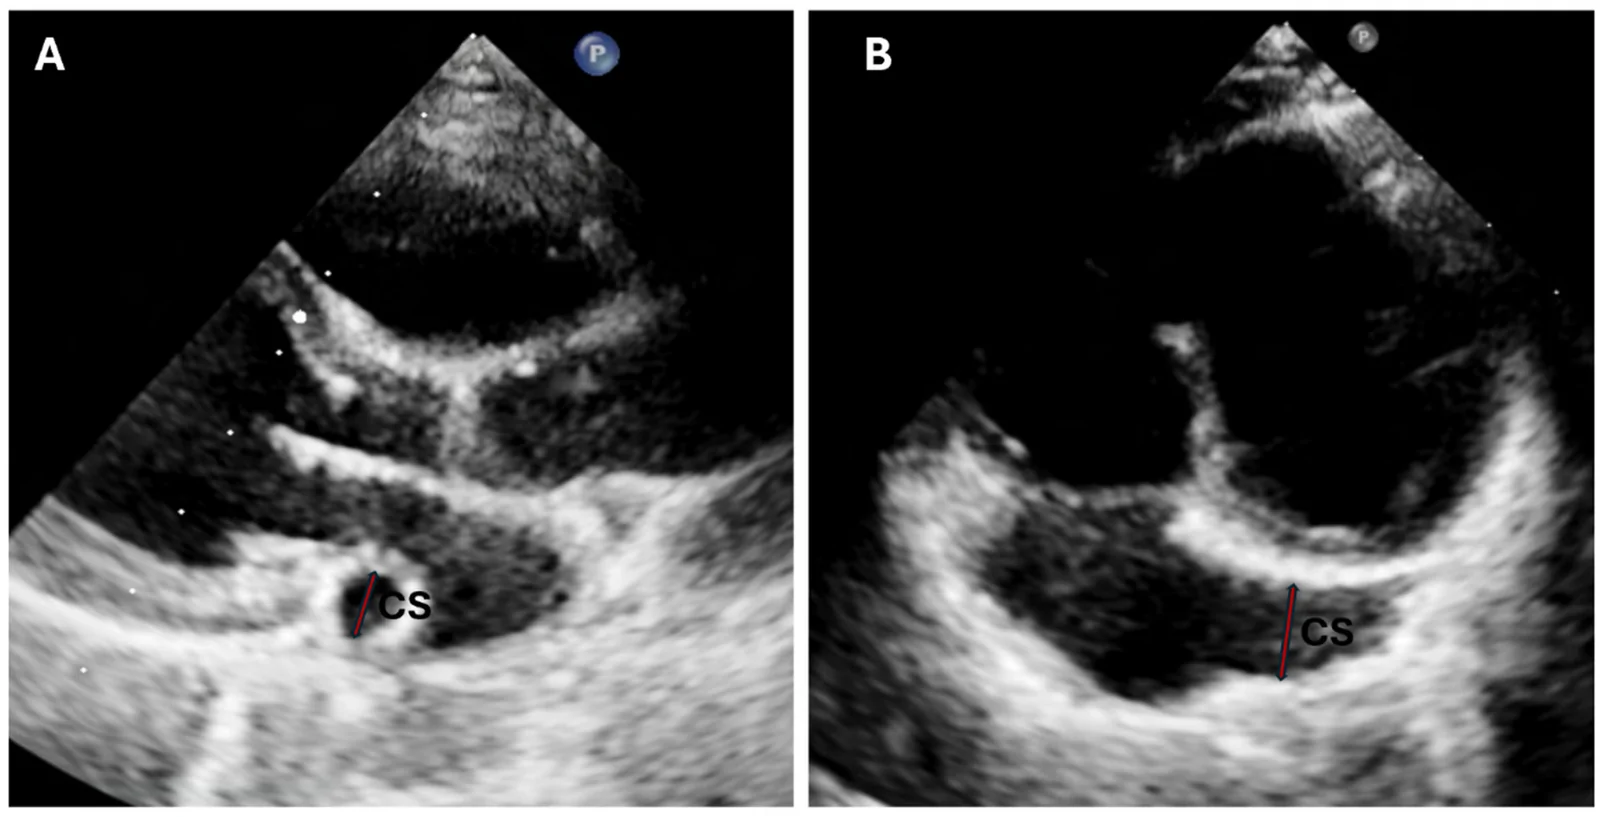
Echocardiographic visualization of the coronary sinus in a patient with persistent left superior vena cava (PLSVC). (A). Parasternal long-axis view demonstrating measurement of the coronary sinus diameter. (B). Apical four-chamber view with posterior tilt demonstrating measurement of the coronary sinus diameter. The arrows indicate the coronary sinus diameter measurement to the figure caption.
